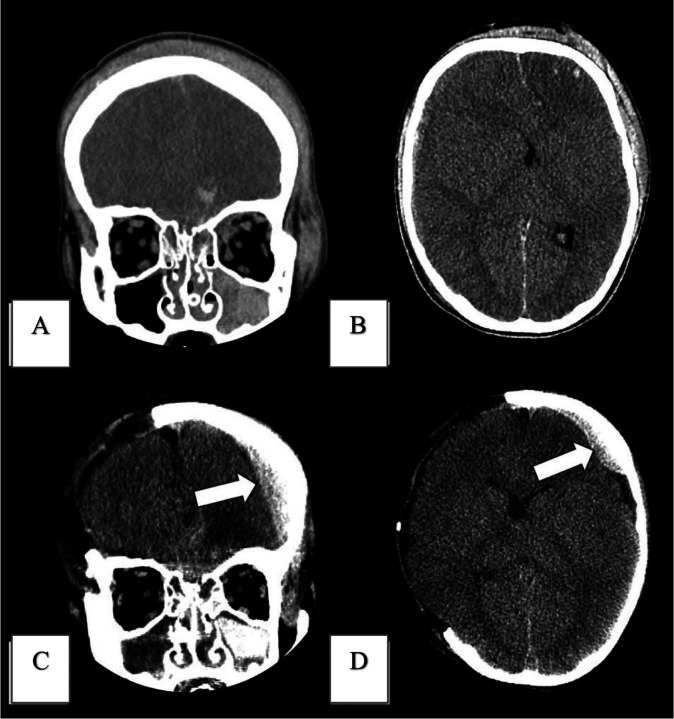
Computed tomograms of the patient’s brain at admission, A (frontal section), B (axial section). Computed tomograms of the patient’s brain after DC. B (frontal section), D (axial section). The arrow shows the development of an acute subdural hematoma in the early postoperative period.
